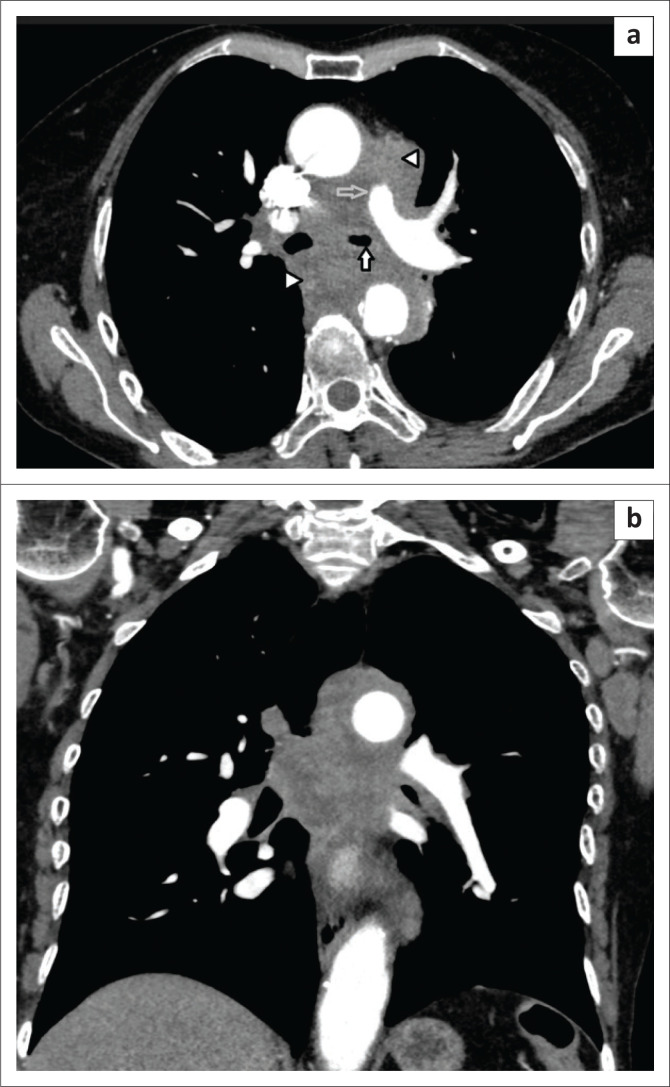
(a) CT chest axial: Confirms extensive hilar and mediastinal mass-nodal complex with necrosis (white arrow heads). The mass encases and narrows the left mainstem bronchus (solid white arrow), main pulmonary trunk (grey arrow), and (b) coronal reconstruction shows trans-spatial extent of the mass.
